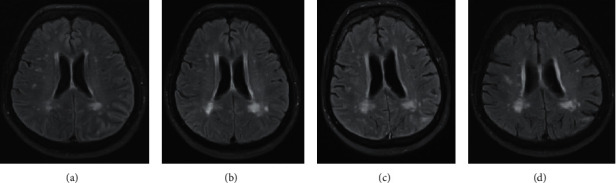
FLAIR images (a–d) showing progressive diffuse signal abnormality involving the white matter of both cerebral hemispheres over 7-year period (a–c) with gradual resolving after steroid treatment (d). (a) June 2012. (b) October 2012. (c) July 2019. (d) February 2020.
